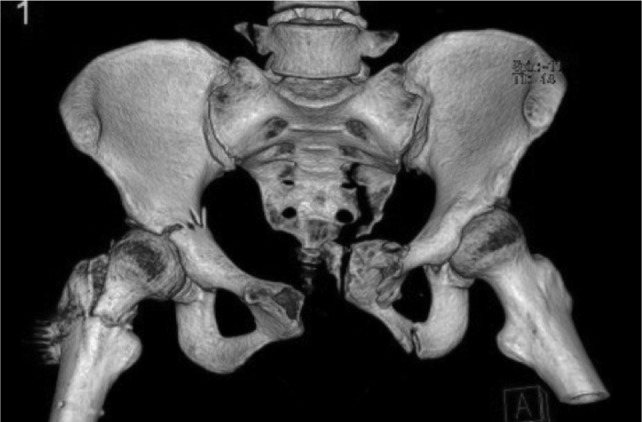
3D Computed tomography (CT imaging) revealed right bicolumnar acetabulum fracture, fracture left superior and inferior pubic rami, fracture right neck of femur.
